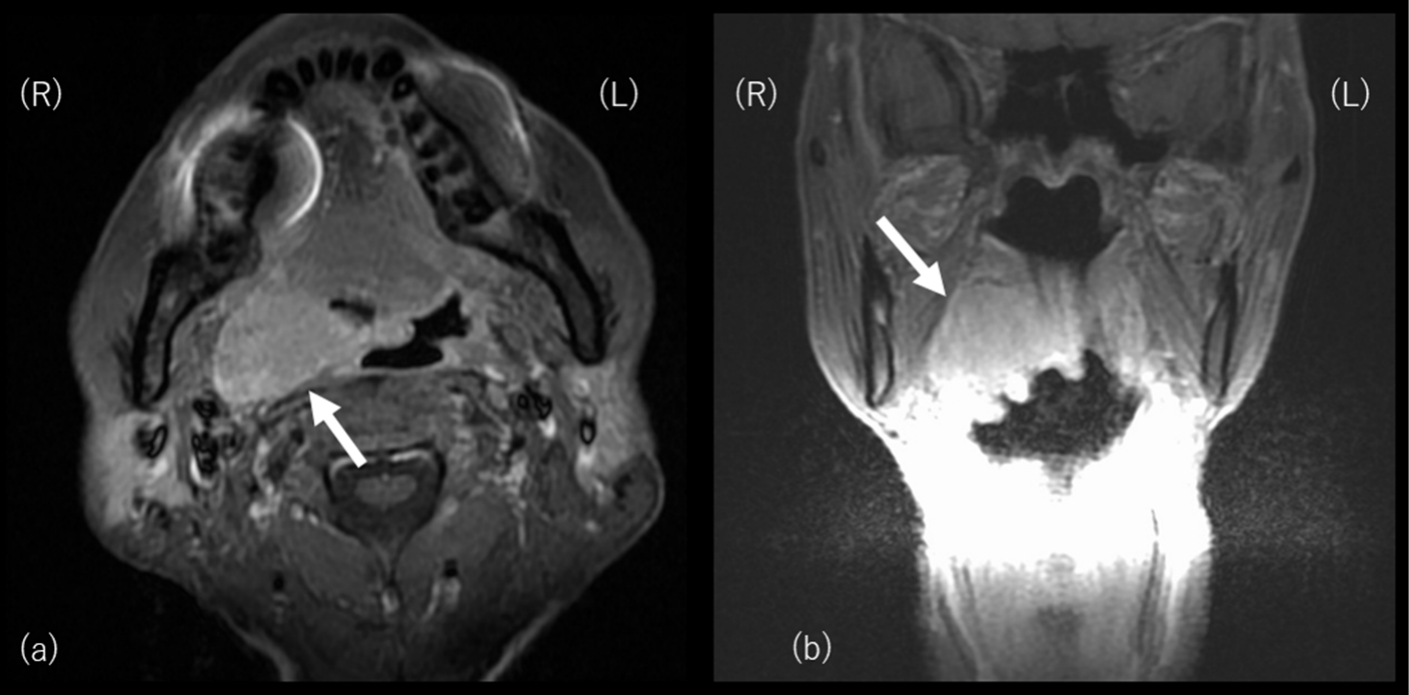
MR images of oropharyngeal squamous cell carcinoma in the right tonsil before primary surgery. Figure 1 (a) and (b) shows an axial and sagittal image of the primary tumor (white arrow).
